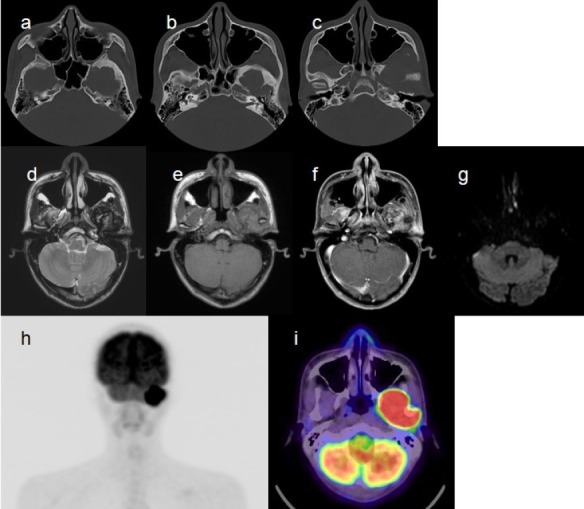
Imaging findings of case number 2. Bone-window CT images (a-c), MRI (d, T2-weighted image; e, T1-weighted image; f, gadolinium contrast-enhanced T1 weighted image; g, diffusion-weighted image), and ^18^F-FDG PET/CT (h, maximum-intensity-projection image; i, fused PET/CT image at the delayed phase) are presented. The findings are similar to those of case 1. SUV_max_ values in the early phase (1h) and the delayed phase (2h) were 17.3 and 19.4, respectively; the RI was 12.1%
